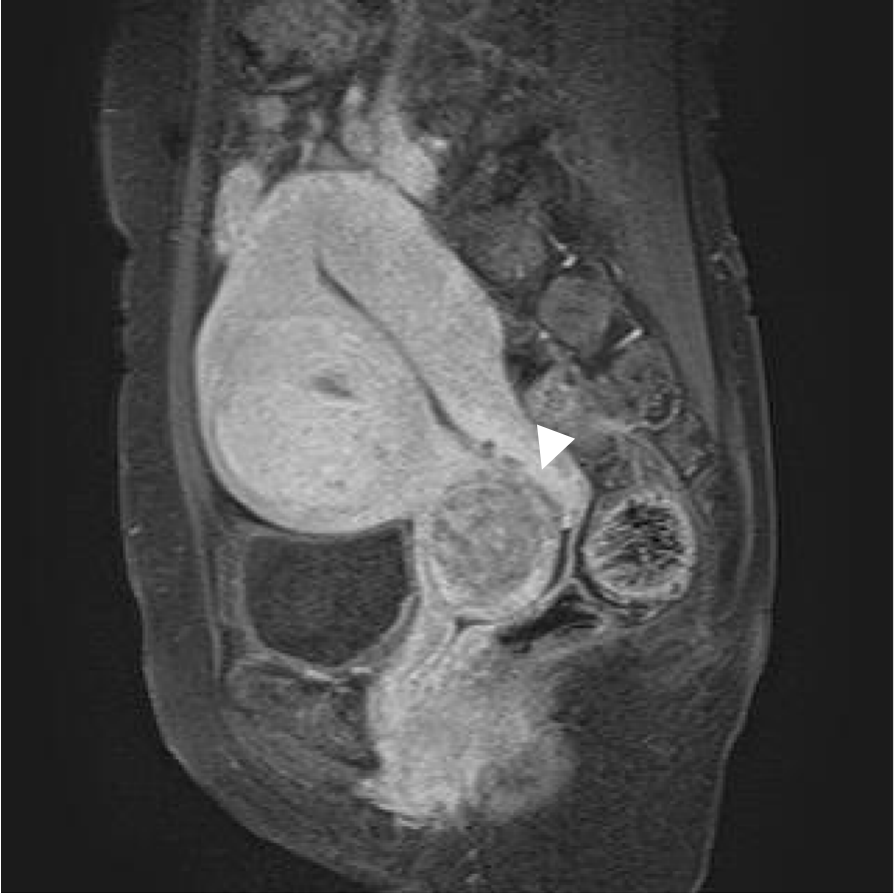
T1-weighted magnetic resonance imaging with fat suppression shows a low-signal lesion measuring approximately 4 cm at the uterine cervix (arrowhead)
